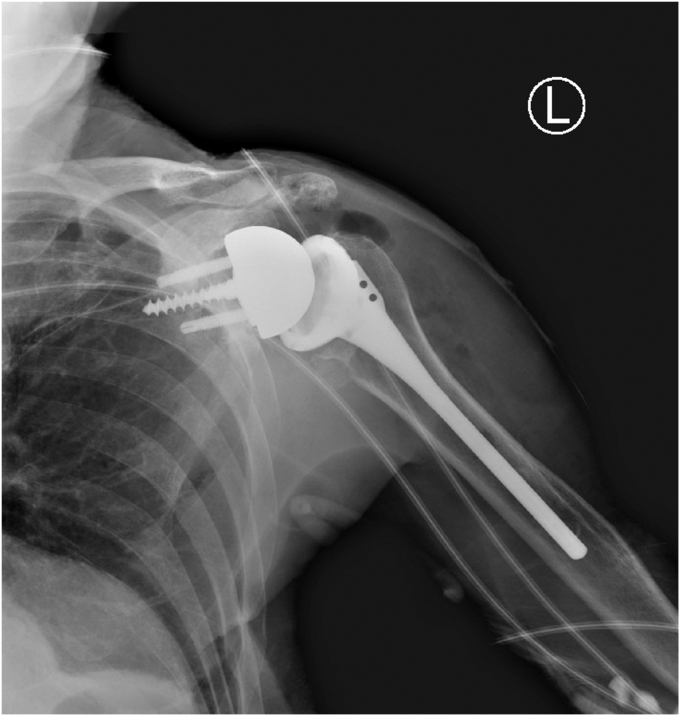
X-ray showing a postoperative image of a reverse total shoulder arthroplasty.
